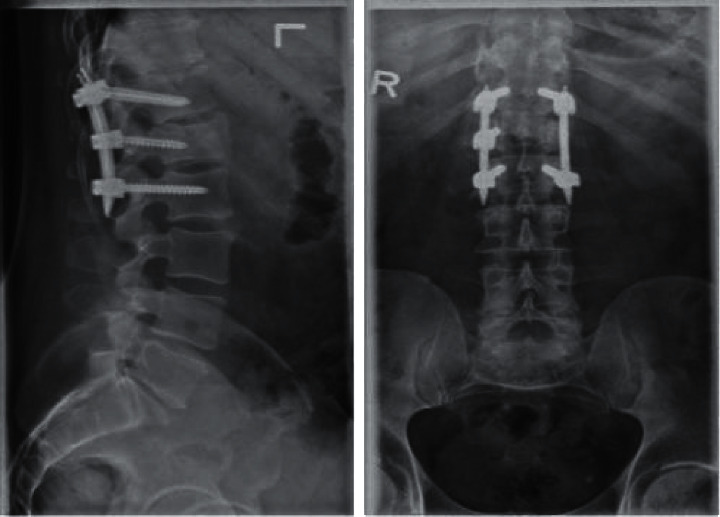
Lateral and AP X-ray of percutaneous stabilization of L1 burst fracture treated percutaneously at T12–L2 at 12-month follow-up.
